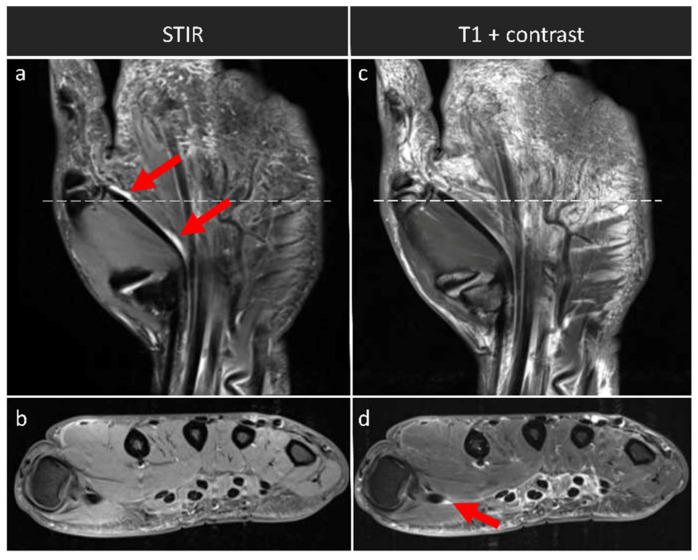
Tenosynovitis in an exemplary patient across the sequences. Coronal STIR (a) and axial T1-weighted sequence with fat suppression, yet without contrast agent application (b) and corresponding coronal (c) and axial (d) T1-weighted post-contrast sequences. Axial images were acquired at the level of distal metacarpal bones (as indicated by the white horizontal lines). Tenosynovitis of the flexor tendon of the first digit can be identified in the STIR sequence (dual red arrows in (a) and in the contrast-enhanced sequences (single red arrow in (d)). Right hand of a 48-year-old male patient.
